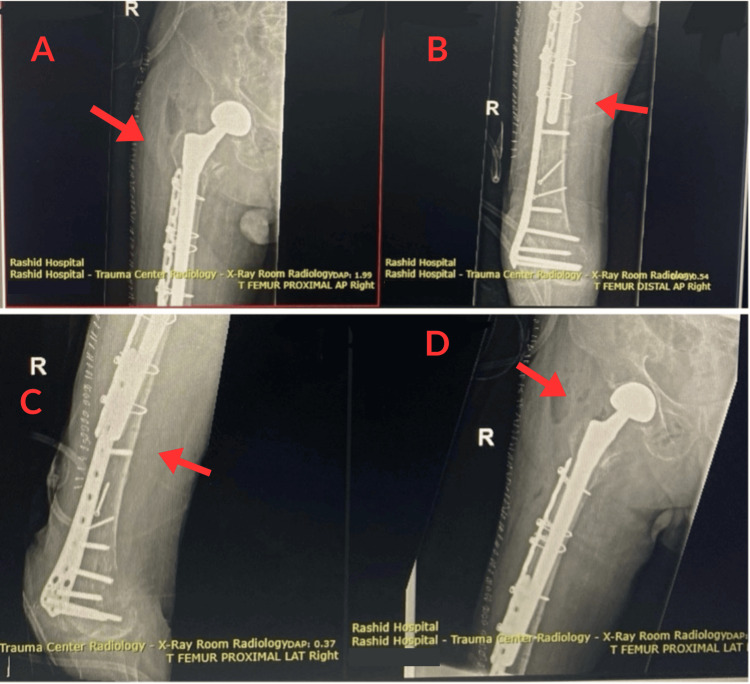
Postoperative right hip X-rays (AP view), 27 June 2025, showing hardware placement and satisfactory alignment after hemiarthroplasty. (A) AP view of the proximal right femur showing the uncemented Wagner SL revision stem and Biolox Delta ceramic head. (B) AP view of the distal right femur demonstrating the lateral locking compression plate extending distally with multiple locking screws. (C) Lateral view of the right femur showing the length and alignment of the locking plate along the femoral shaft. (D) Lateral view focusing on the proximal femur and hip prosthesis, demonstrating proper positioning of the femoral stem and absence of hardware complications.
